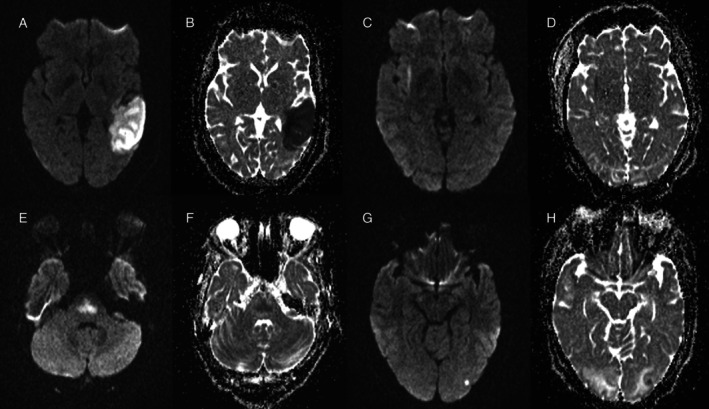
Images of four study participants with infarcts detected on diffusion‐weighted MRI. Images A, C, E and G are from diffusion‐weighted sequences with a *b*‐value of 1000 s/mm^2^. Images B, D, F and H are apparent diffusion coefficient (ADC) maps. Cytotoxic edema in acute infarcts is characterised by high signal intensity on diffusion‐weighted images and low signal intensity in ADC images. Images A and B (participant 1) show a large infarct in the posterior part of the left medial cerebral artery territory. Images C and D (participant 2) show a linear infarct in the right external capsule. Images E and F (participant 3) show an infarct in the pons, and images G and H (participant 4) show a small, rounded infarct in the left occipital cortex. Subcortical high signal in image H corresponds to areas of vasogenic oedema related to posterior reversible encephalopathy syndrome (PRES).
